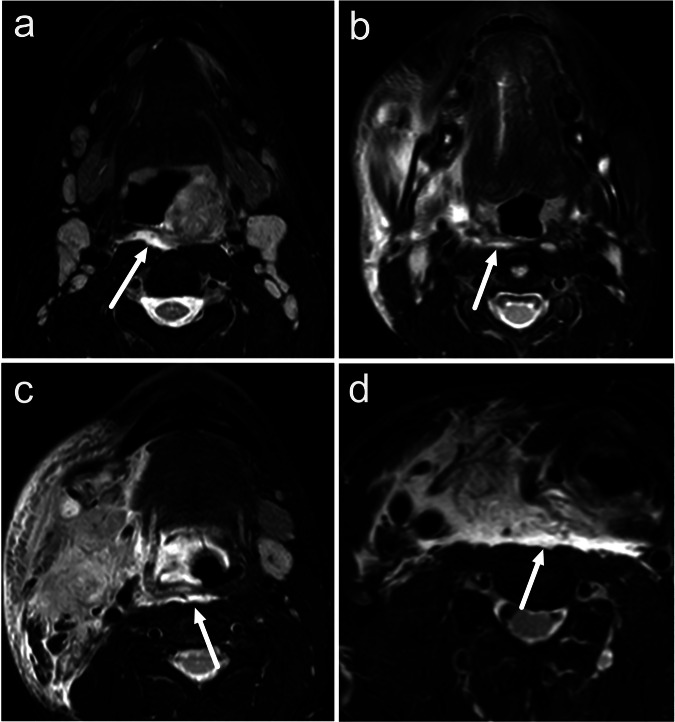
Examples of retropharyngeal edema (arrows) on axial T2-weighted Dixon water-only images of four different patients (a, b, c, d) with acute neck infections
